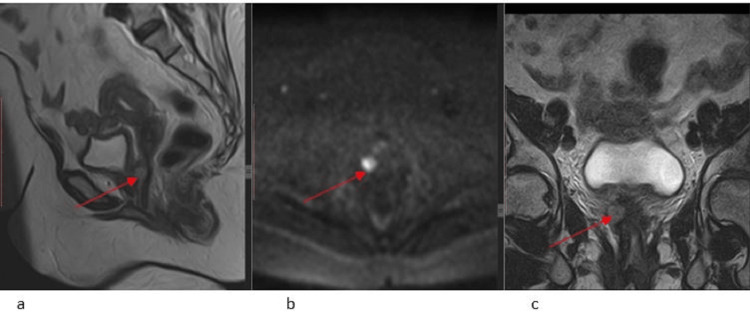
Follow-up MRI pelvis after nine months of completion of therapy demonstrates post-chemoradiation changes with interval development of a circumscribed hyperintense T2 signal area along the right anterolateral aspect of the upper third of the vagina on sagittal T2W1 (6a), corresponding to diffusion restriction on DWI (6b), concerning for recurrent disease DWI: diffusion-weighted imaging
